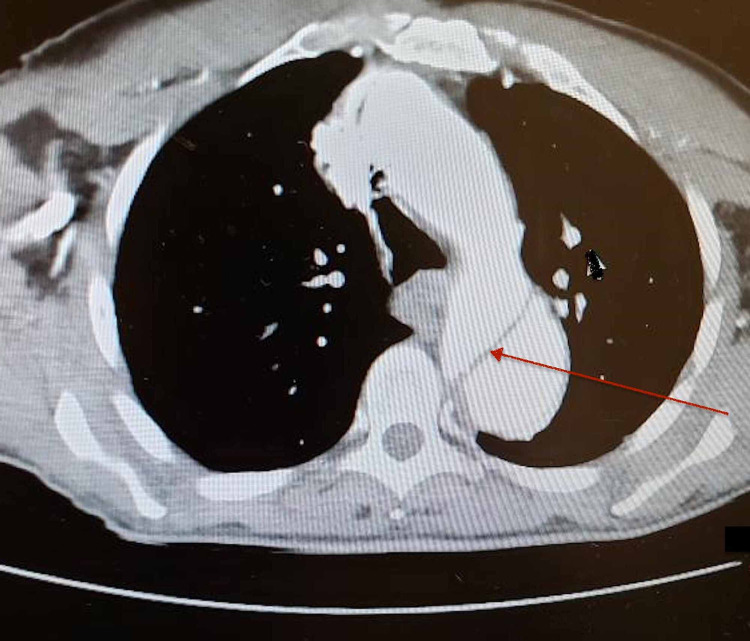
CT chest showing aortic dissection with an intimal flap.
